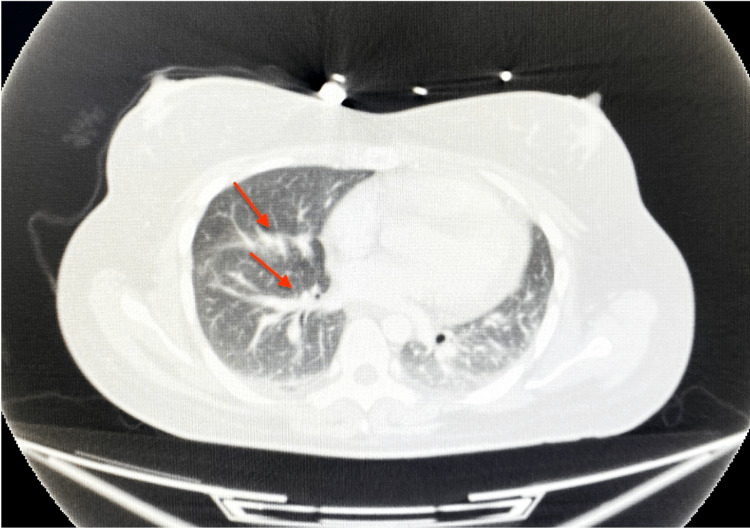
Chest CT (computed tomography) scan
